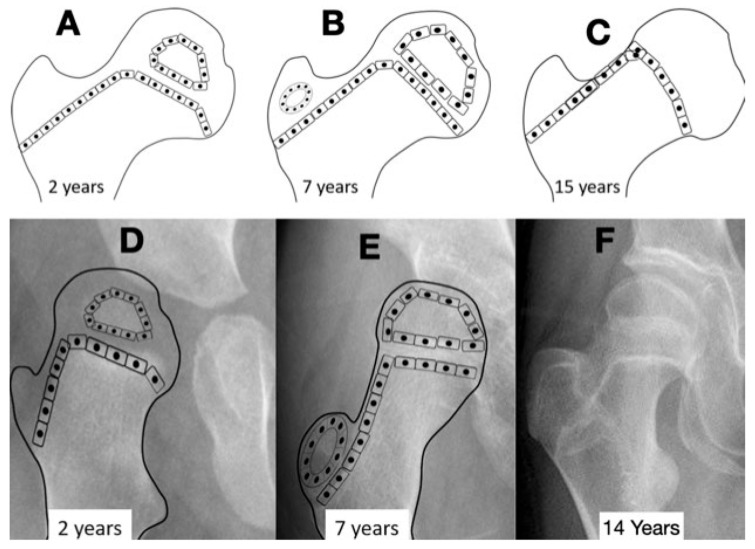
The normal growth plate of the developing femur in a 2-year-old child (A), a 7-year-old child (B), and an adolescent (C) has apophyseal and epiphyseal segments that are in continuity with the intermediate epiphysis. The triangular-shaped epiphyseal plate has differential growth with a relatively slower growth of the apophyseal side compared with the epiphyseal side, creating a more sharply angled growth plate. This difference in apophyseal versus epiphyseal growth in children with cerebral palsy compared with the typically developing child explains the observed difference in proximal femoral shapes (D,E). The intermediate growth plate contributes to longitudinal femoral growth and width of the femoral neck as shown in this girl, Gross Motor Function Classification System level V, who was treated with pamidronate at age 8 years, leaving a dense line; this radiograph was taken at the completion of growth at age 14 years (F). The shape of the growth plate is unchanged until the end of growth. The acetabulum, however, has increased lateral ossific growth as the ring apophysis is ossified. This lateral growth, combined with the femoral head having symmetric medial and lateral growths, has reduced the migration percentage from 28% at age 8 years to 20% at age 14 years (F).
